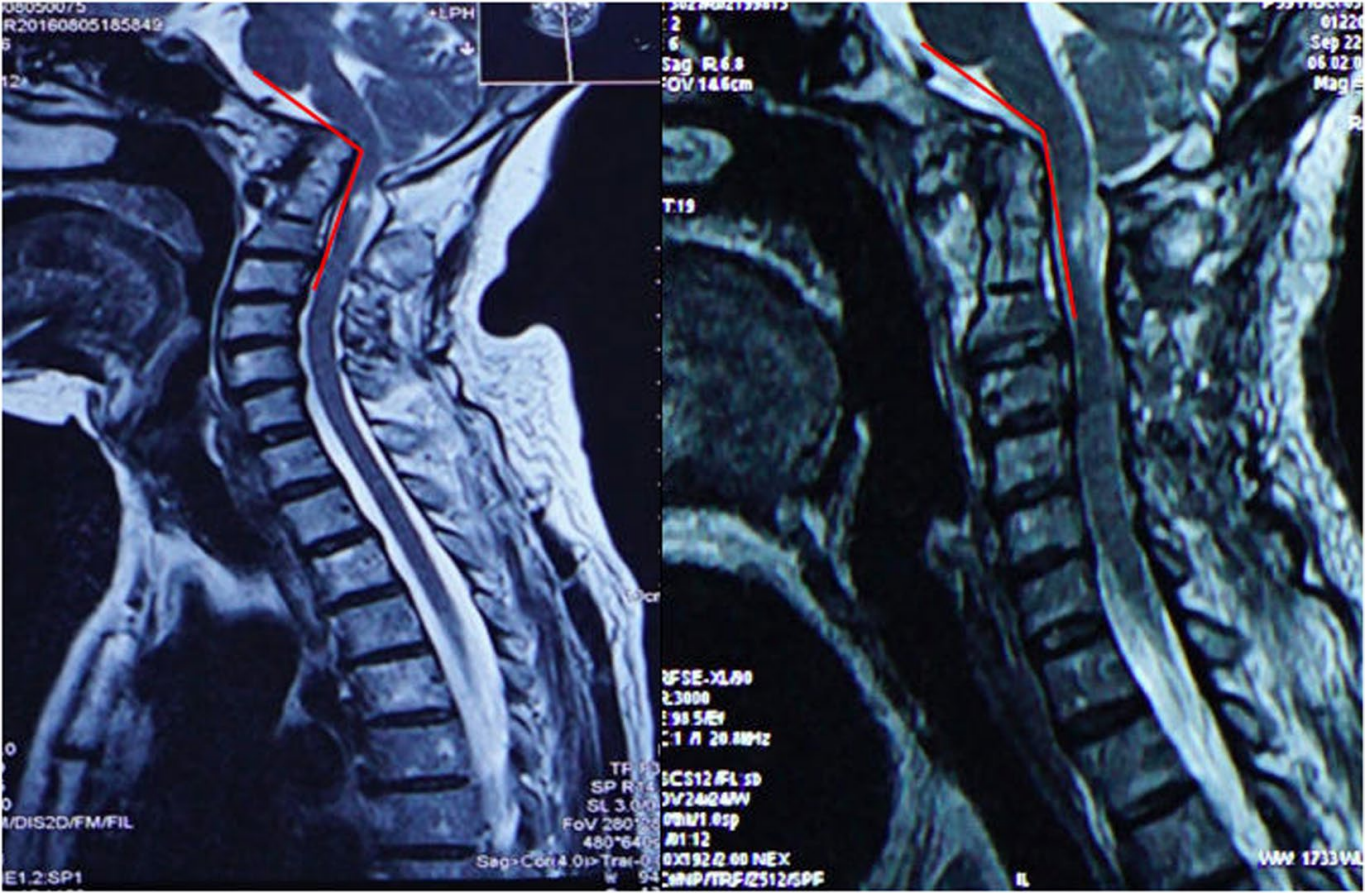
The CMA is defined as the included angle between the line parallel to ventral side of medulla oblongata and the line parallel to the ventral side of the upper cervical cord in MRI of cervical spine. An MRI sagittal imaging showed that CMA had changed pre-(left) and post-operation (right).
